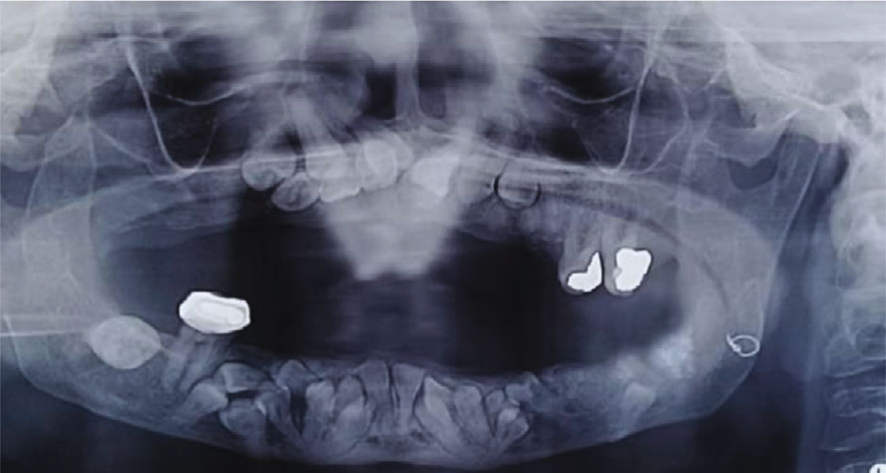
Panoramic radiograph 3 months after teeth extraction.
